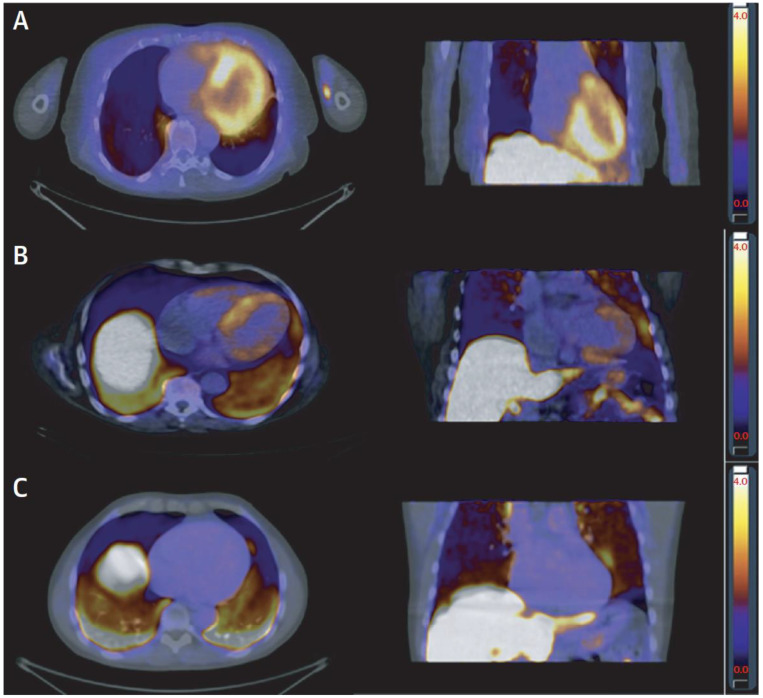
Examples of summed PET images taken 10–20 min after injection of ^11^C-PIB (see below for abbreviations). The signal represents myocardium/blood SUV ratio, and the color scale ranges from black (SUV ratio 0) to white (SUV ratio 4.0). Transaxial and coronal view of (A) a patient with AL amyloidosis and high degree of ^11^C-PIB uptake in the left ventricle wall and septum; (B) a patient with ATTR amyloidosis and moderate degree of ^11^C-PIB uptake in the cardiac walls, most apparent in the septum; and (C) a healthy volunteer with no myocardial ^11^C-PIB uptake. All subjects show high signal in the liver, which is due to normal metabolization of ^11^C-PIB, and in the lungs, which is most likely caused by tracer lipophilicity. ATTR, transthyretin amyloidosis; PET, positron emission tomography; PIB, Pittsburgh compound B; SUV, standardized uptake value. Reproduced, with permission, from [50].
